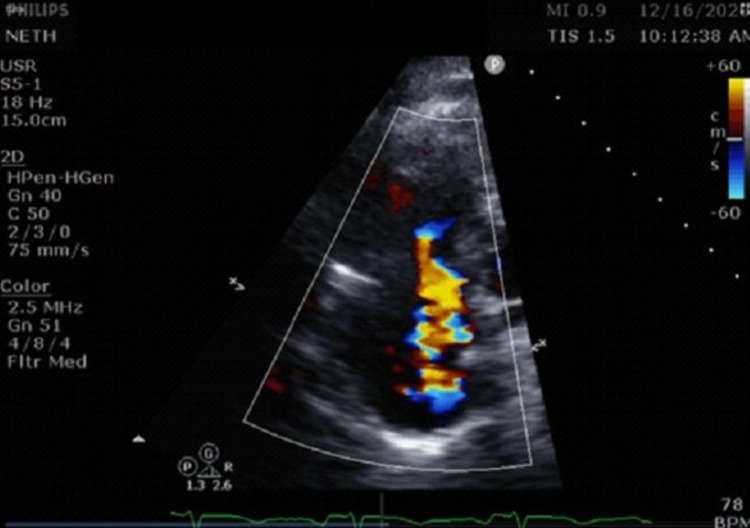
Transthoracic four-chamber view showing severe tricuspid regurgitation.
